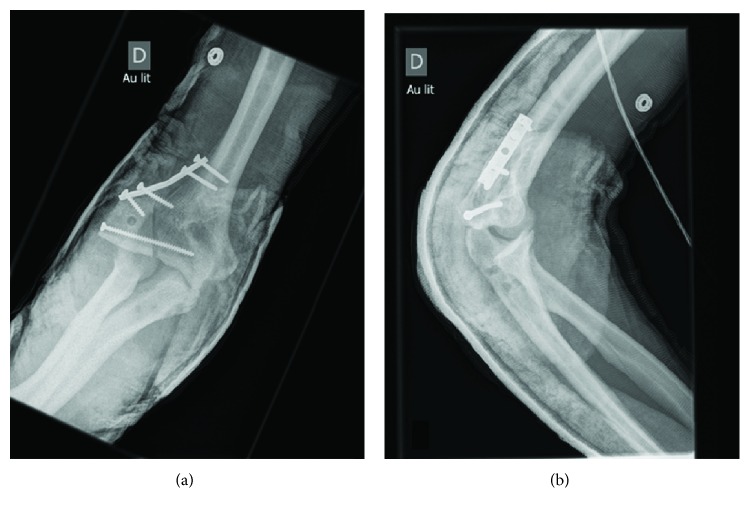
(a) Anteroposterior and (B) lateral 14 days postoperative X-rays of the right elbow.
